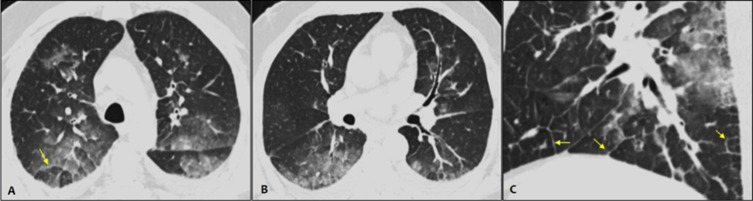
axial (A and B) and aggrandized right lower lobe sagittal (C) CT images without intravenous contrast showing bilateral and central ground-glass with broncho vascular bundle and interlobular septal thickening (arrows)
